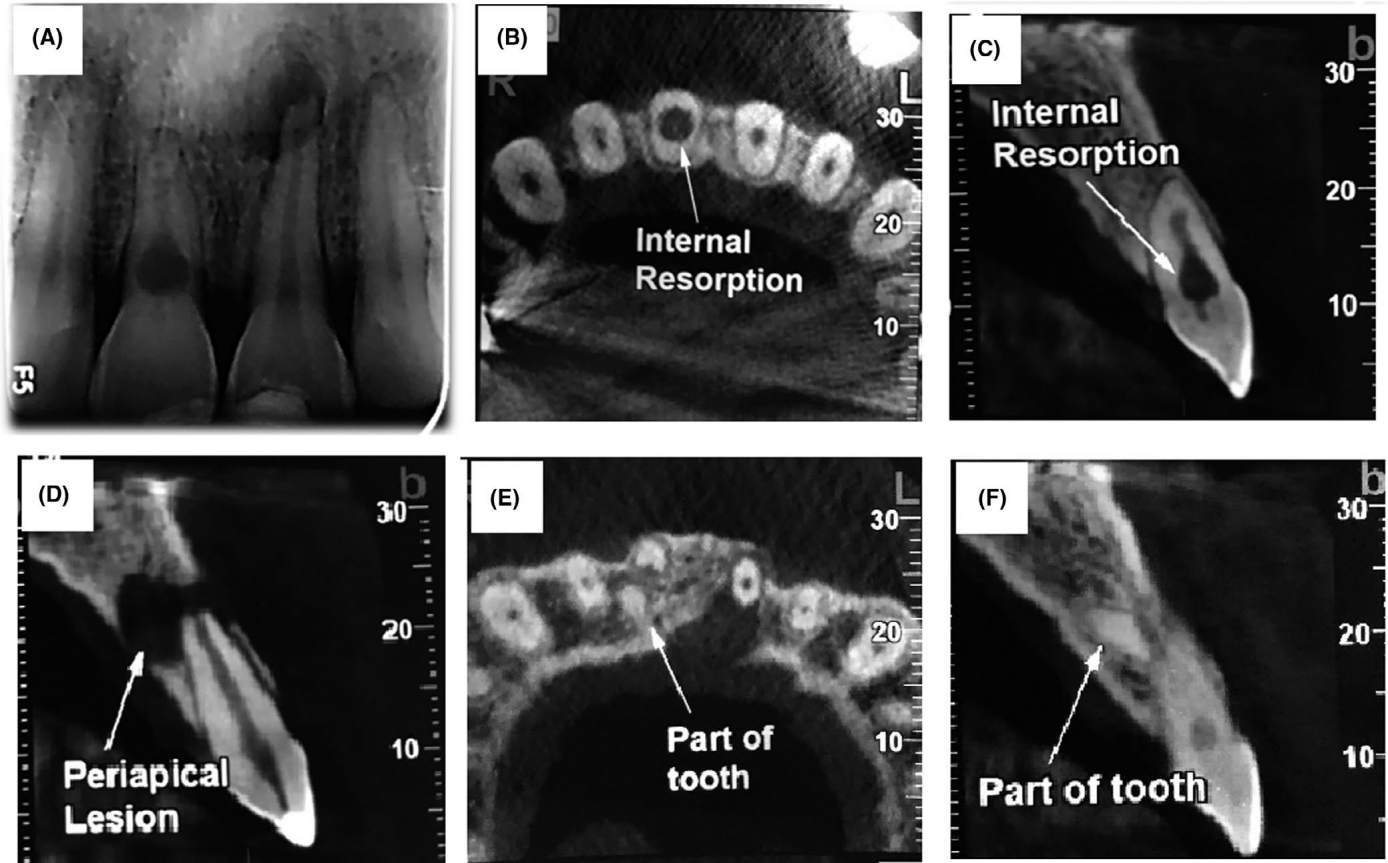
Preoperative radiography (A) and CBCT from axial (B, E) and sagittal view (C, D, F)
